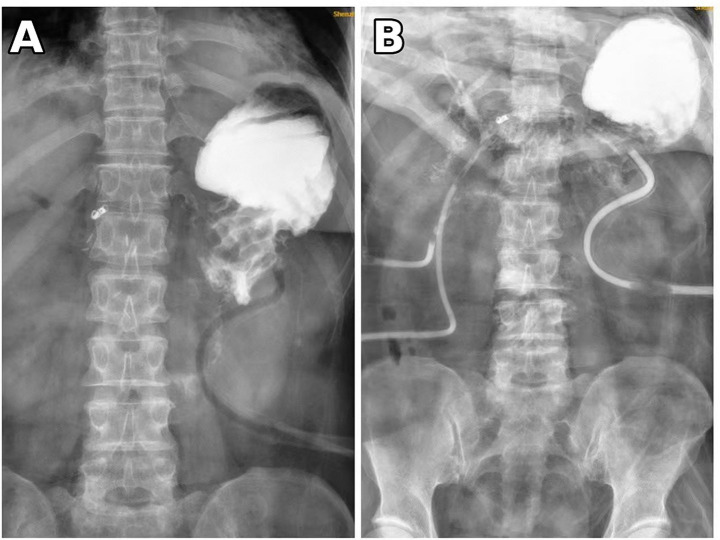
Pre-intervention contrast study. (A) Abdominal radiograph obtained 30 min after contrast instillation through the nasogastric tube, showing retention of contrast in the remnant stomach with limited downstream passage. (B) Radiograph obtained 3.5 h after contrast instillation, showing persistent gastric retention and delayed emptying into the efferent limb.
